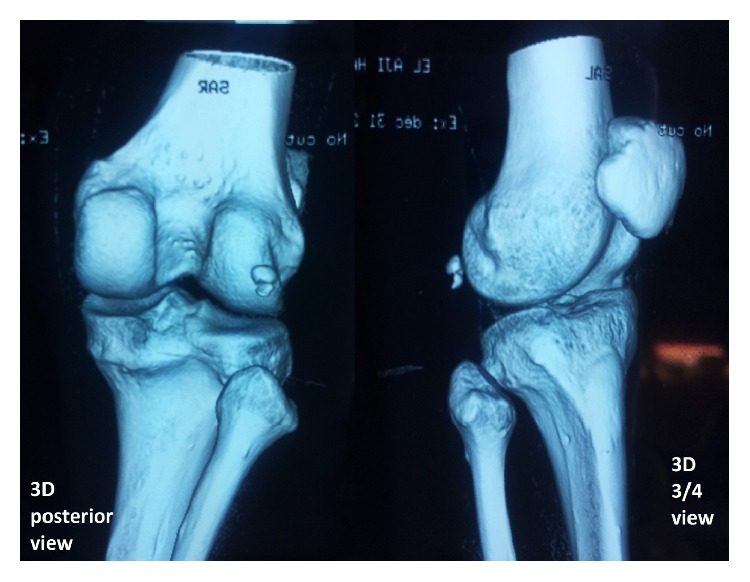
3D-CT reconstruction revealed a fracture of the fabella.
